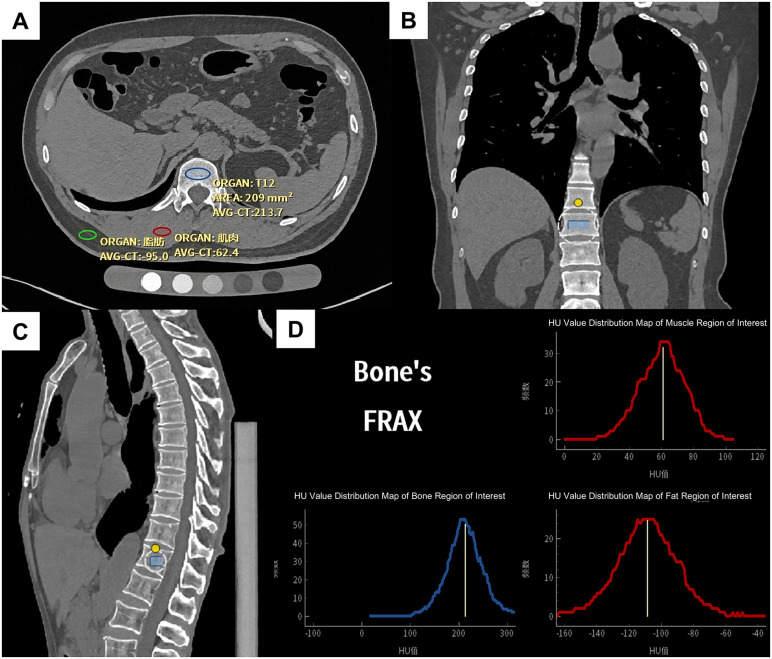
Selection process for the PL-QCT ROI (A-D). (A) Axial CT image showing the vertebral body (blue ellipse) and the reference ROIs for fat (green ellipse) and muscle (red ellipse) tissues. (B) Coronal and (C) Sagittal reconstructed images. (D) Histograms of the HU value distributions for the three ROIs: the top red histogram corresponds to the muscle ROI, the left blue histogram corresponds to the vertebral body ROI, and the right red histogram corresponds to the fat ROI.
